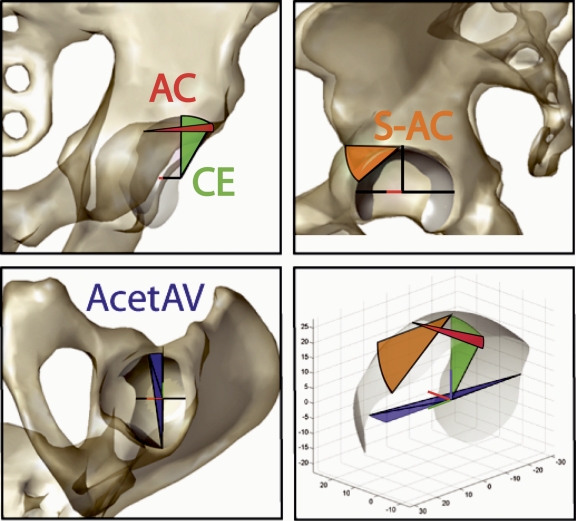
Angles describing the orientation of the acetabulum and femoral coverage are calculated from a 3D segmented model of the pelvis in frontal, sagittal, and transverse planes emulating those traditionally measured from radiographs or reformatted CT scans. In the frontal plane (top left), the AC angle measures the obliqueness of the acetabular roof between the medial aspect of the sourcil, the lateral edge of the acetabular rim, and a horizontal line. The CE angle measures the coverage of the lateral edge of the acetabulum with respect to the center of the femoral head (not shown), and may have a negative value in severely dysplastic cases. The orientation of the acetabular cup (S-AC angle) in the sagittal plane (top right) is measured from the most superior aspect of the acetabular roof to the most anterior aspect with respect to horizontal. The anteversion (AcetAV angle) is measured on a transverse plane (bottom left) viewed inferior to superior using a line created between the posterior and anterior rim of the acetabulum. The angle is measured with respect to a line normal to the two femoral head centers (intercapital centerline). The angle measurements help to characterize the orientation of the load-bearing surface of the hip joint in 3 dimensions (bottom right) during realignment (axis scale in mm; viewed isometric from anterio-lateral-superior).
